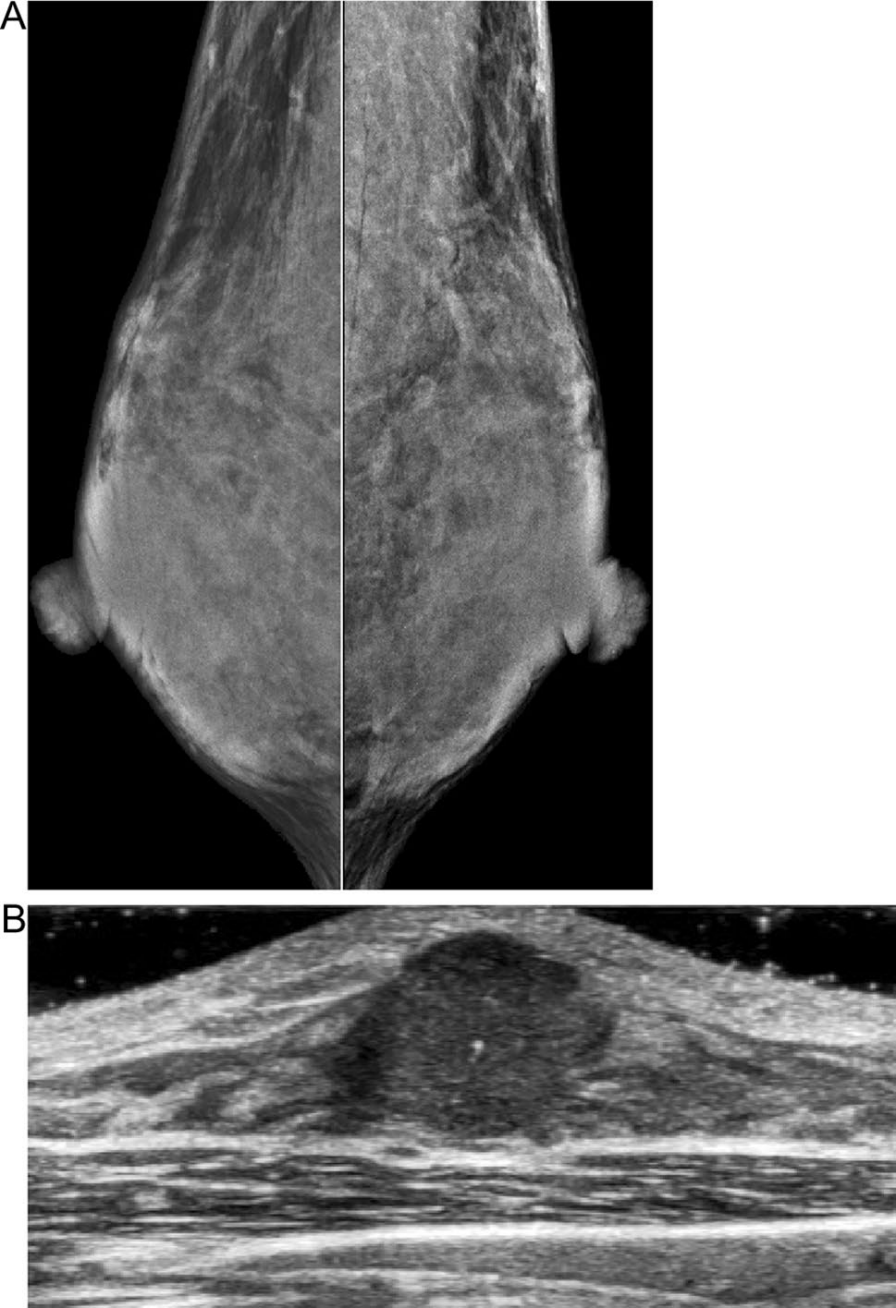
Mammographically occult invasive ductal carcinoma measuring 19 mm was detected by ultrasonography in a 47-year-old woman with extremely dense breasts. A There are no mammographic abnormalities due to the masking effect. B The gray-scale breast ultrasonography image shows a hypoechoic mass with microlobulated margins
